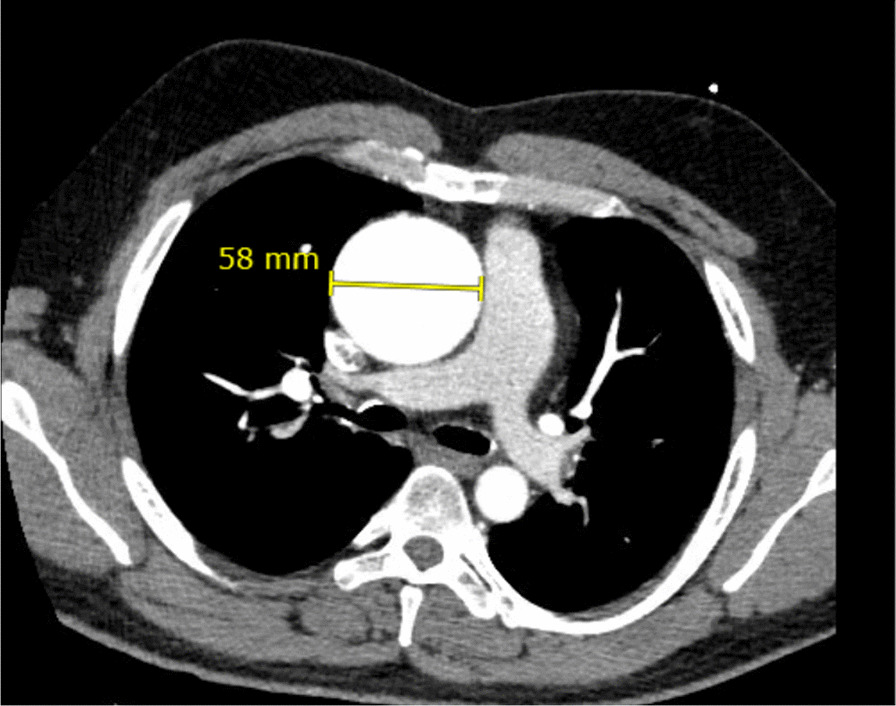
CTA chest axial cut demonstrating stable fusiform aneurysmal dilation of ascending thoracic aorta measuring 5.8 cm in maximum transverse diameter, mildly increased from previous CT 4 mo prior. No evidence of aortic dissection or hematoma
